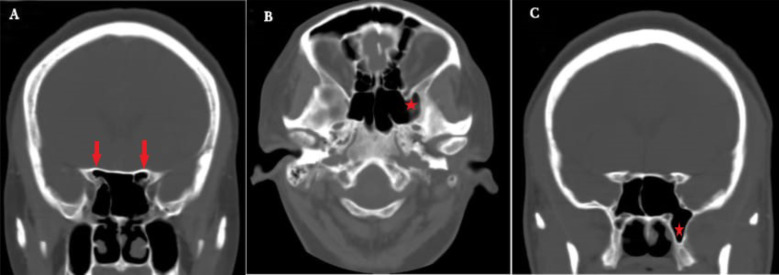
CT images showing extension of pneumatization of the sphenoid sinus. A. Bilateral anterior clinoid process pneumatization B. Left greater wing of sphenoid pneumatization C. Left pterygoid process pneumatization.
